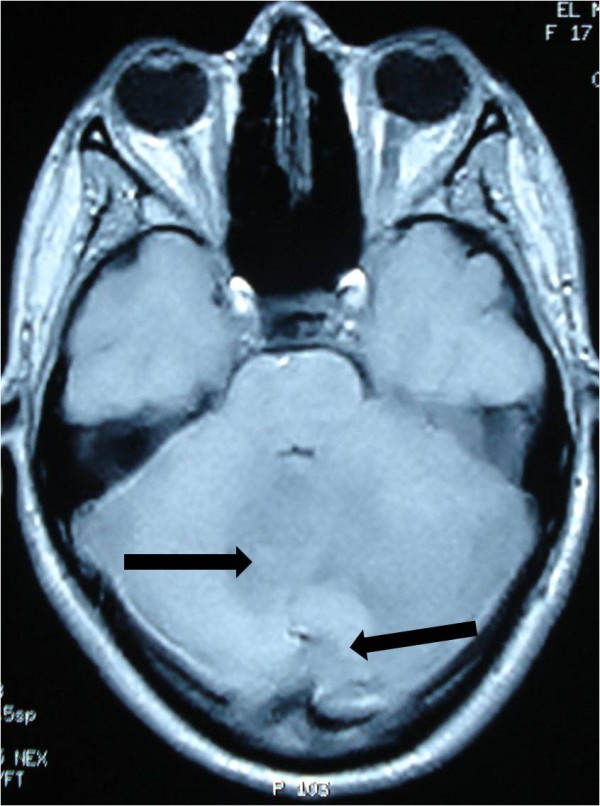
Cranial magnetic resonance imaging of irregular contours of the mass lesions that are in contact with the fourth ventricle and the confluence of sinus (arrow). The processes are heterogeneous on T1-weighted imaging.
